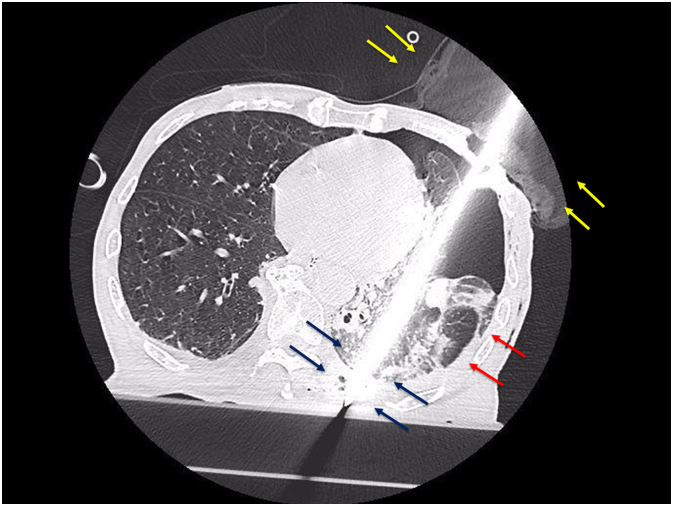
Chest CT showing penetration of the lower left lobe that reached the left dorsal chest wall. The yellow arrows show the knife secured with a towel or tape. The black arrows show the knife penetrating to the dorsal muscle layer of the 9th intercostal space. The red arrows show that there is no massive pleural effusion. (For interpretation of the references to colour in this figure legend, the reader is referred to the web version of this article.)
